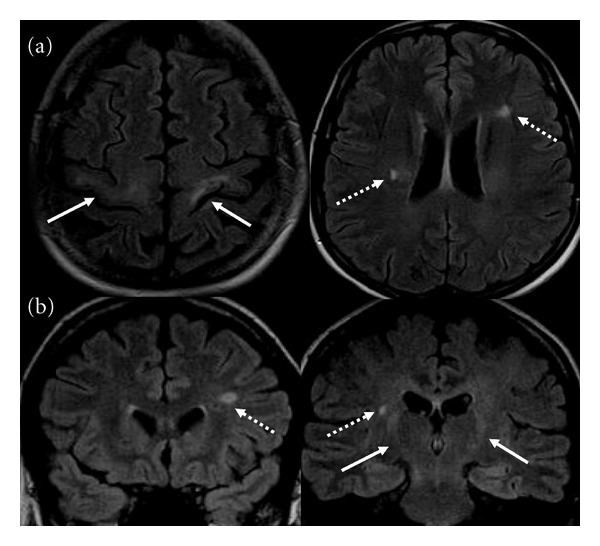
Axial (a) and coronal (b) FLAIR MR images showing typical hyperintensities of pyramidal tracts (solid line), from motor cortices to bulbar pyramids. In this context, periventricular and juxtacortical lesions, hyperintense in T2 and FLAIR (dashed line), were observed.
